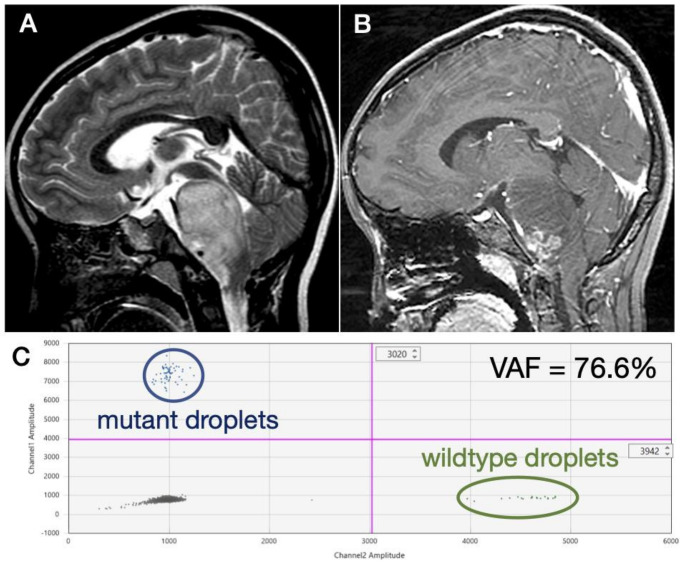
Representative case in which the *H3F3A* mutation was detected in CSF obtained by lumbar puncture at presentation. MR images show (A) a diffuse hyperintensity of the pons on T2-weighted images and (B) an exophytic growth at the lower pons and encasement of vertebral arteries by enhancing tumor on post-contrast images. (C) Many mutant and wildtype droplets were detected, and the variant allele frequency was 76.6%.
